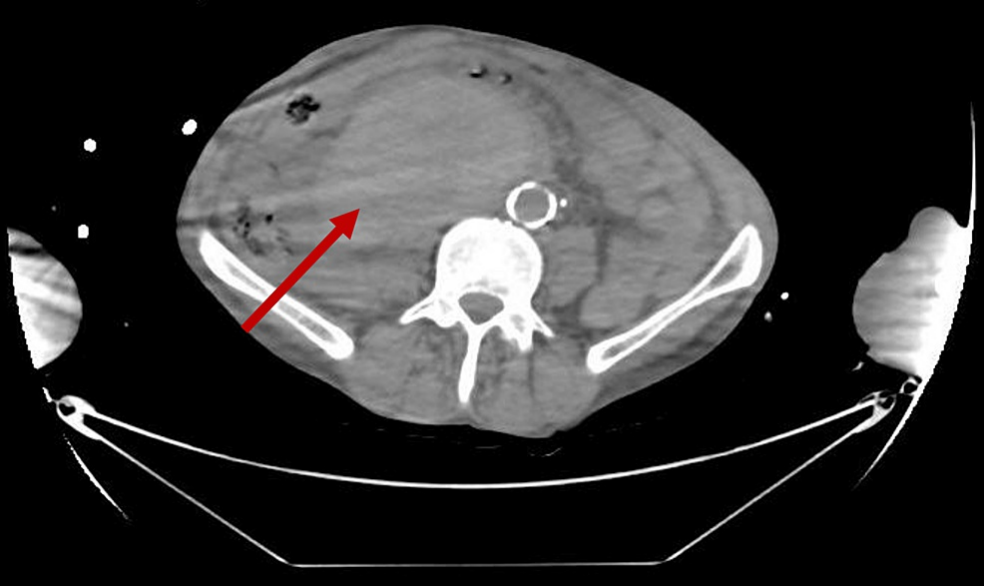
CT Abdomen/Pelvis (axial view) The red arrow is showing the haematoma
